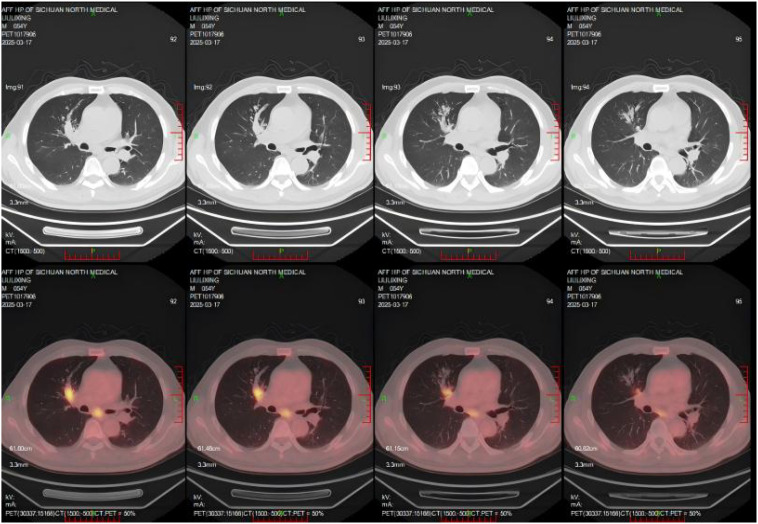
PET-CT findings demonstrated bronchial truncation in the anterior segment of the right upper lobe, local patchy-nodular soft-tissue density with increased density in the distal lung field, mild bronchiectasis in some bronchi, and abnormally elevated FDG metabolism. Enlarged lymph nodes with abnormally elevated FDG metabolism were also observed in the pretracheal, retrocaval, and subcarinal regions.
